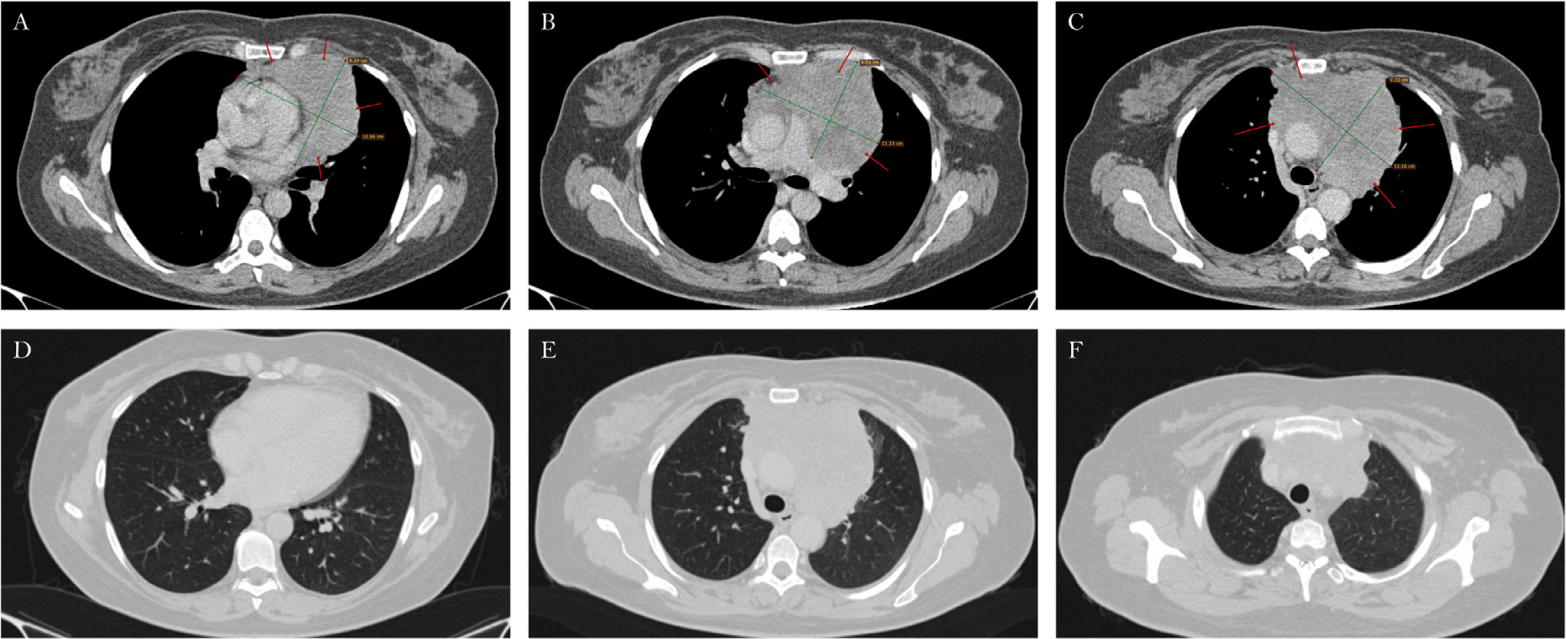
Initial CT findings in axial view. A Thymic formation with hetero-dense characteristics is visible in the mediastinum (A, B, C). There is no visible metastatic disease in the lung window.
